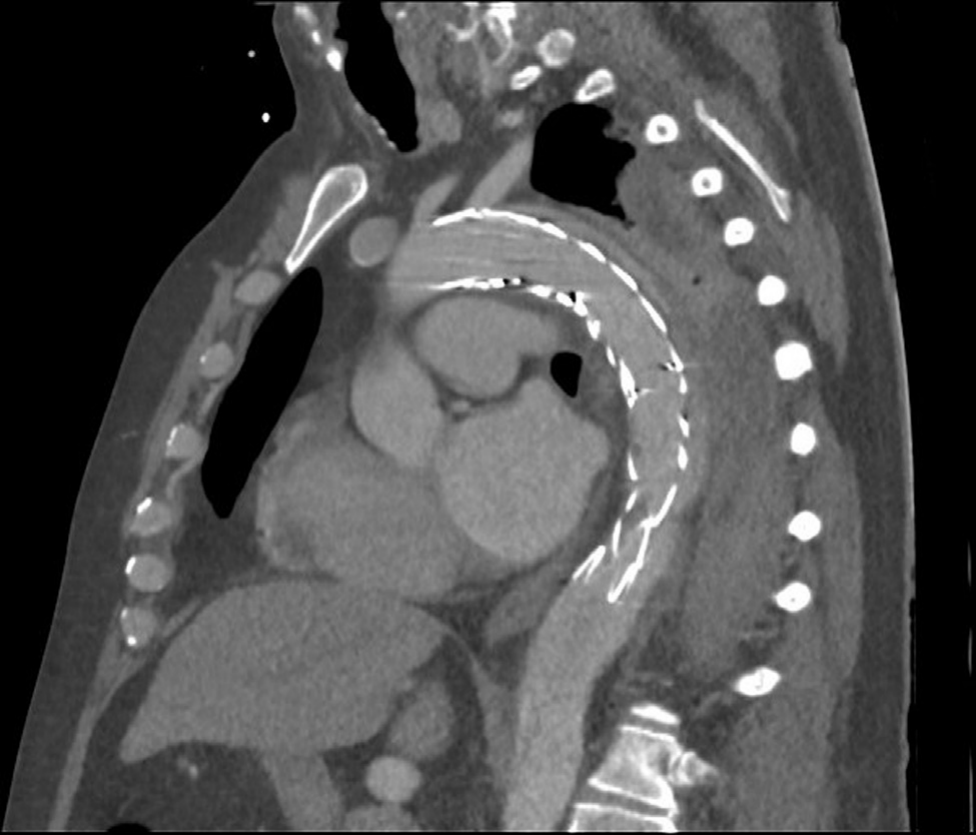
Computed tomography angiography showing a correctly placed aortic endoprosthesis in the absence of complications.
